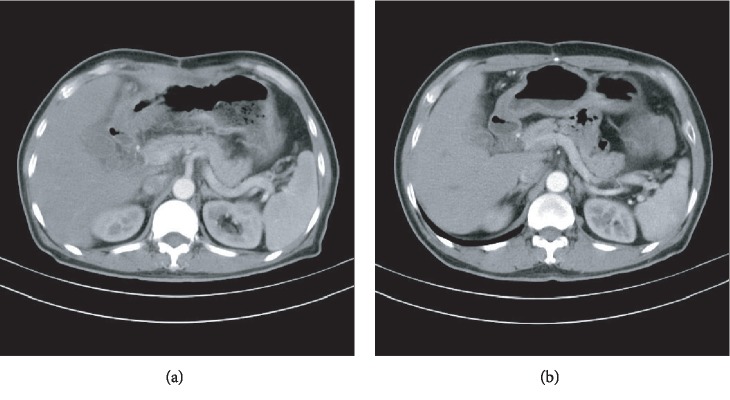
CT scan images of the patient: (a) after 3 cycles of EOX regimen and (b) after 12 cycles of FOLFIRI regimen.
